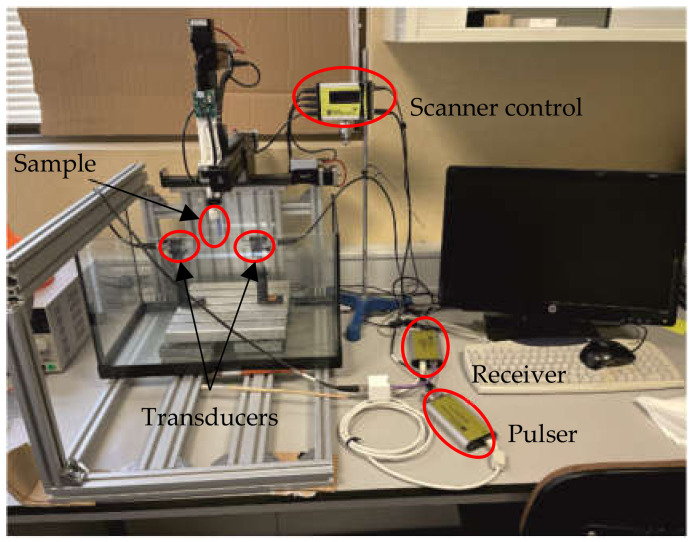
Experimental setup for ultrasonic measurements.
